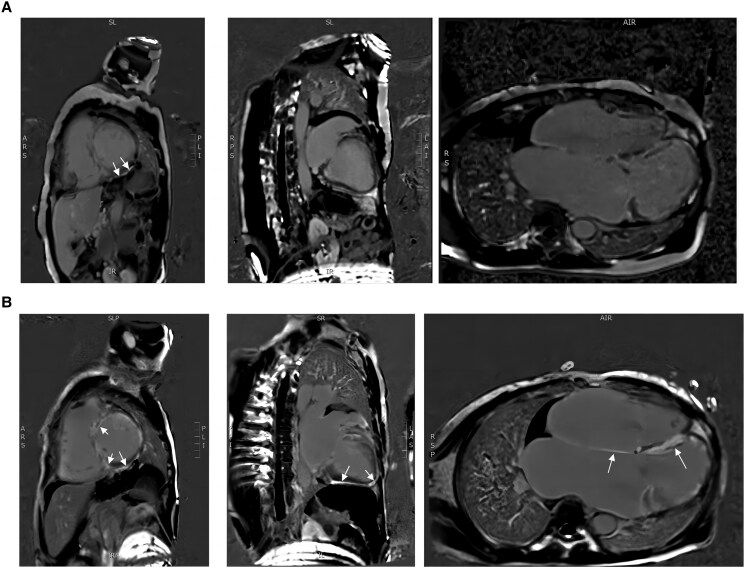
(*A*) Cardiac MRI of the patient in 2021. (*B*) Cardiac MRI of the patient in 2022. In the patient's cardiac MRI in 2021 (*A*), late gadolinium enhancement imaging of the myocardium revealed delayed enhancement of the interventricular septum and the epicardium of the left ventricular inferior wall. However, in 2022, cardiac MRI showed widespread delayed enhancement of all walls of the left ventricle, indicating a progression of the disease.
